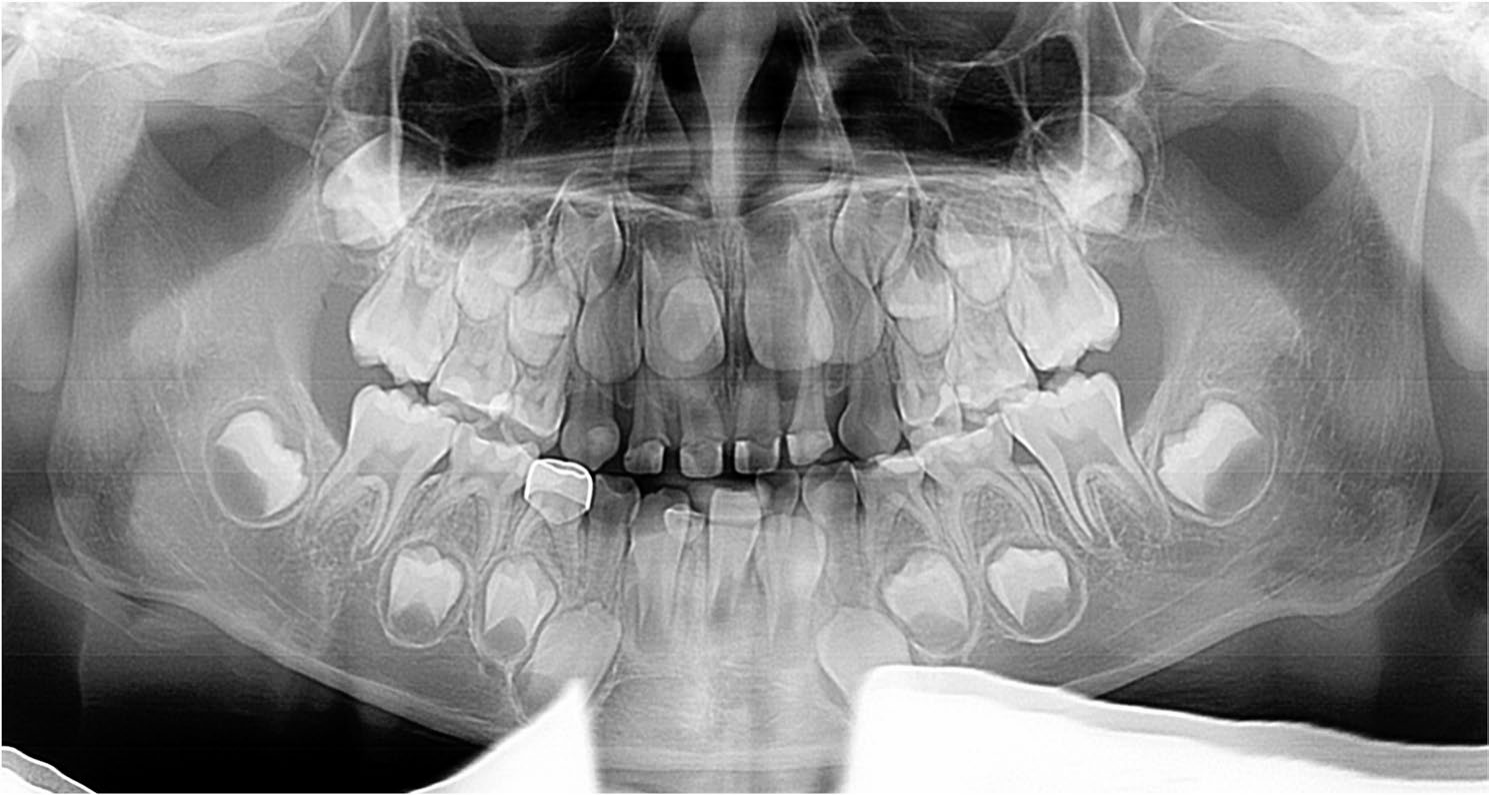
Panoramic radiograph of a 7-year-old male showing congenitally missing right mandibular lateral incisor, 1 supernumerary tooth located in maxillary anterior region
